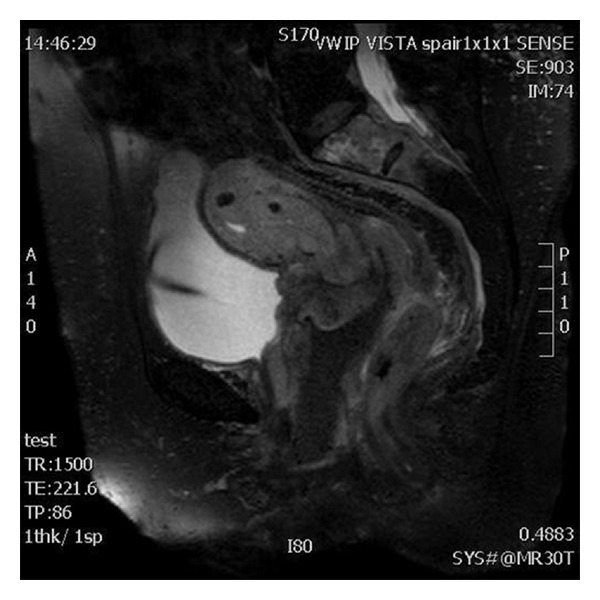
3D VISTA T2-weighted sagittal MRI images of the pelvis. Images show a locally invasive tumor, with heterogeneous rather high intensity signal, most likely originating from the cervix with transmural invasion of the uterus. Invasion into the anterior part of the vagina, bladder, lumbosacral plexus, sacrum, and mesorectum. (The vagina, low/dark signal, was filled with a mixture of barium and gel at the start of the MRI examination for accurate evaluation of vaginal wall.)
